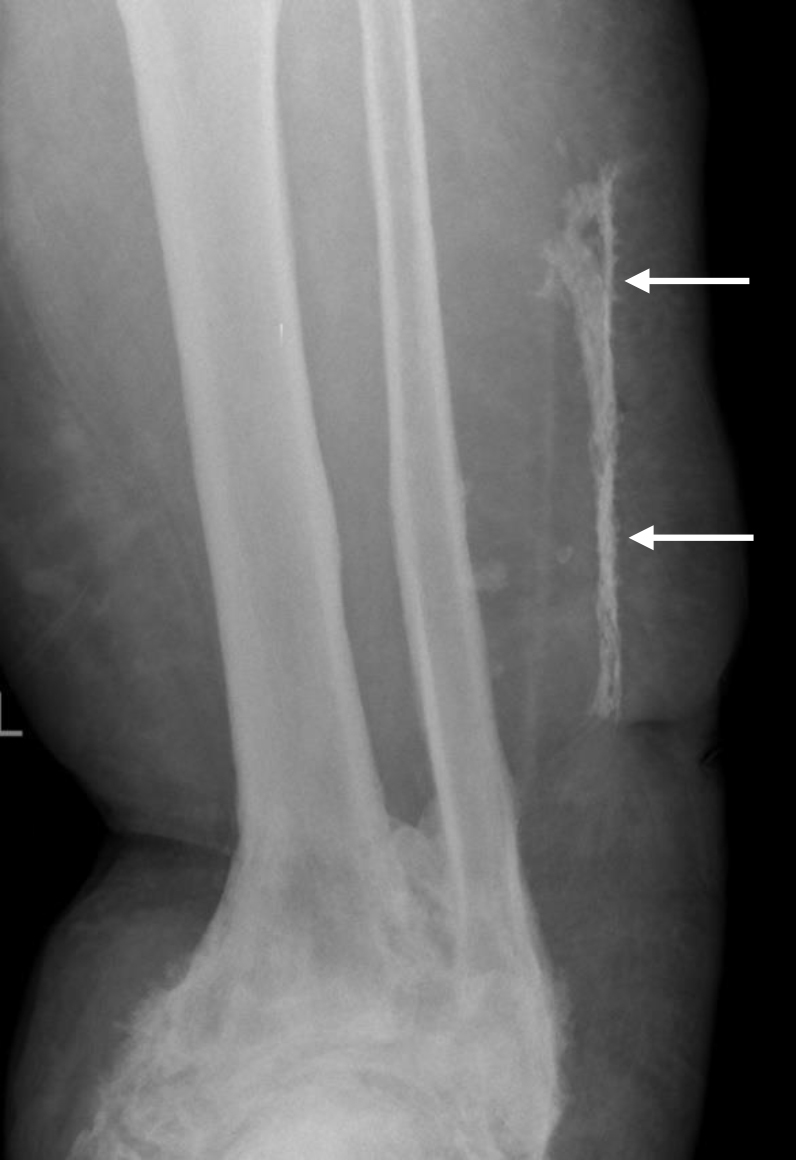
Plain X-ray showed the area of new bone formation in the lateral soft tissues (white arrows), separate from the fibula. The ankle joint has
